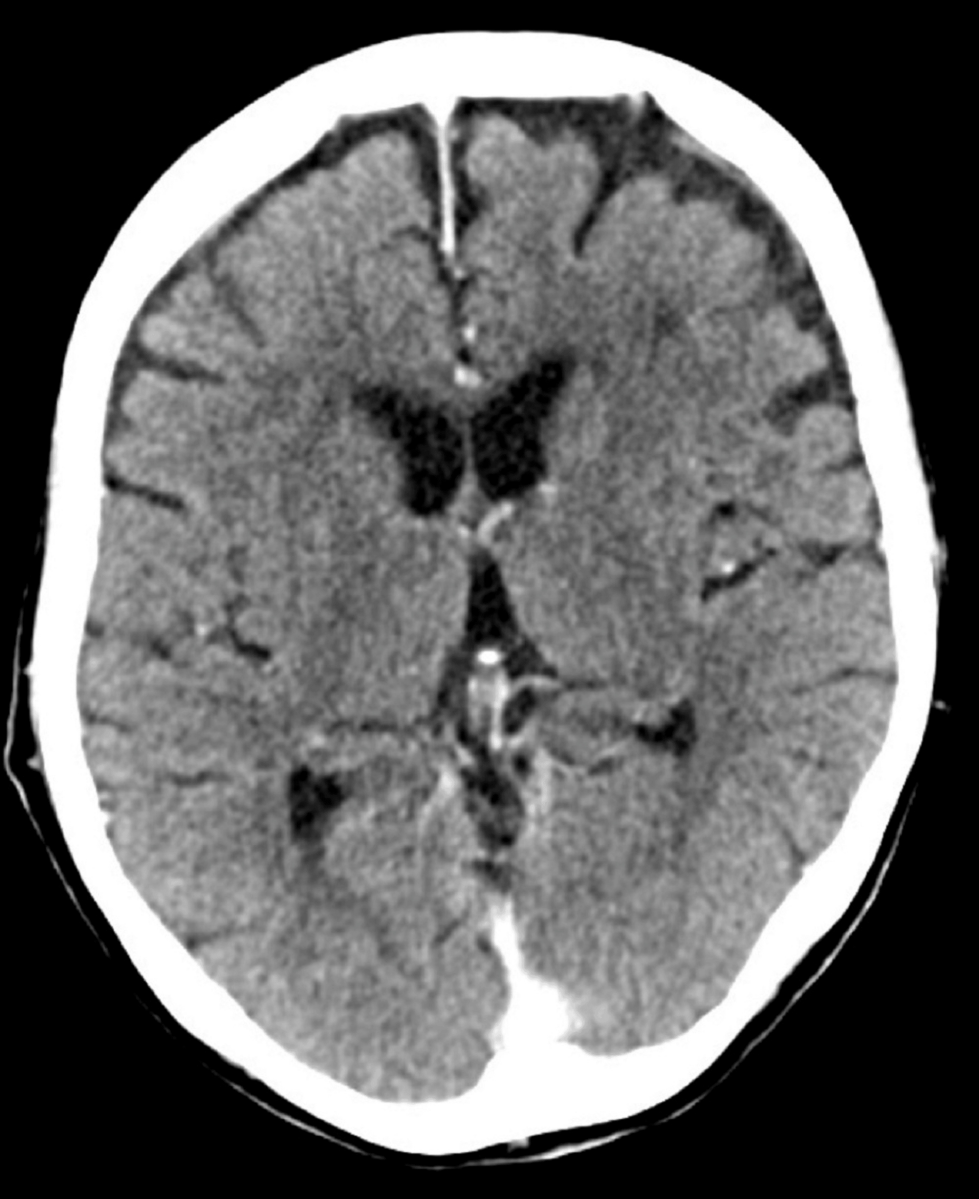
CT of the head with contrast showing no pathological intra- or extra-axial intracranial enhancement and no space-occupying lesion or surface collection CT: computed tomography
